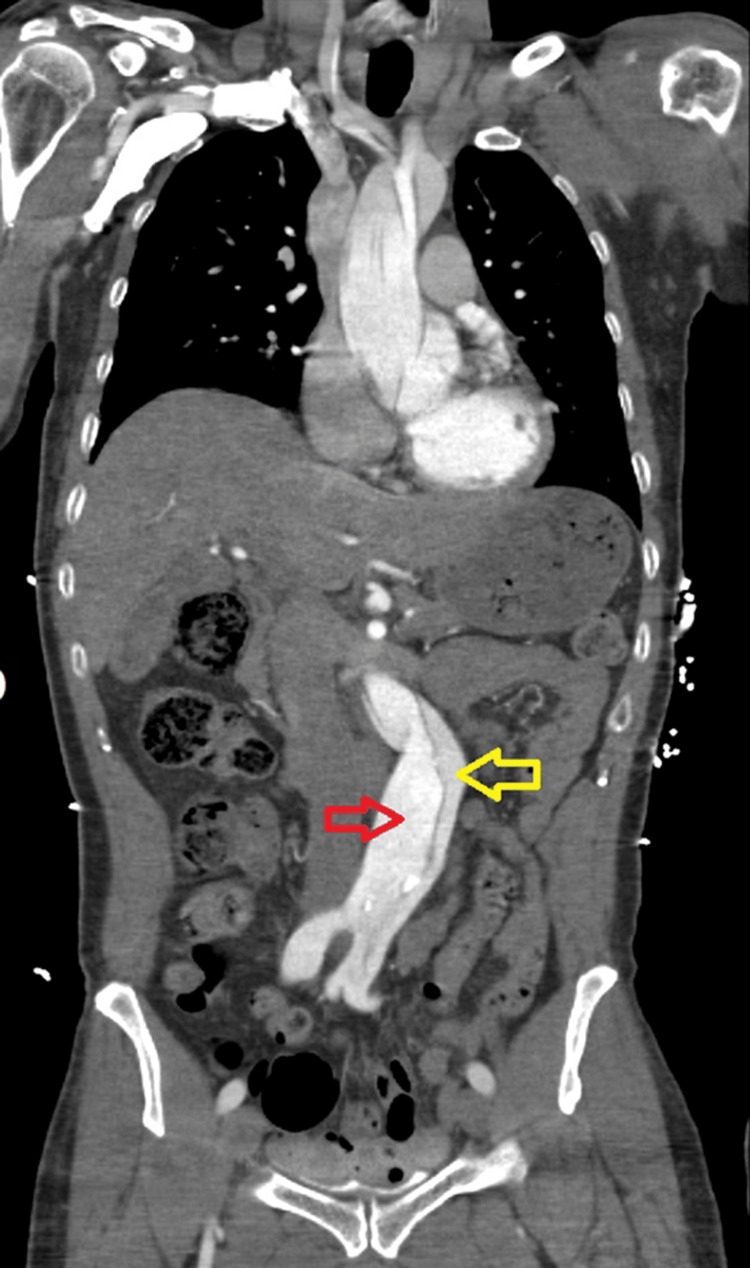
Coronal CT angiography image of the abdominal and thoracic aorta showing a true (red arrow) and false lumen (yellow arrow) with stenosis of the true lumen of the abdominal aorta due to compression from the false one.
